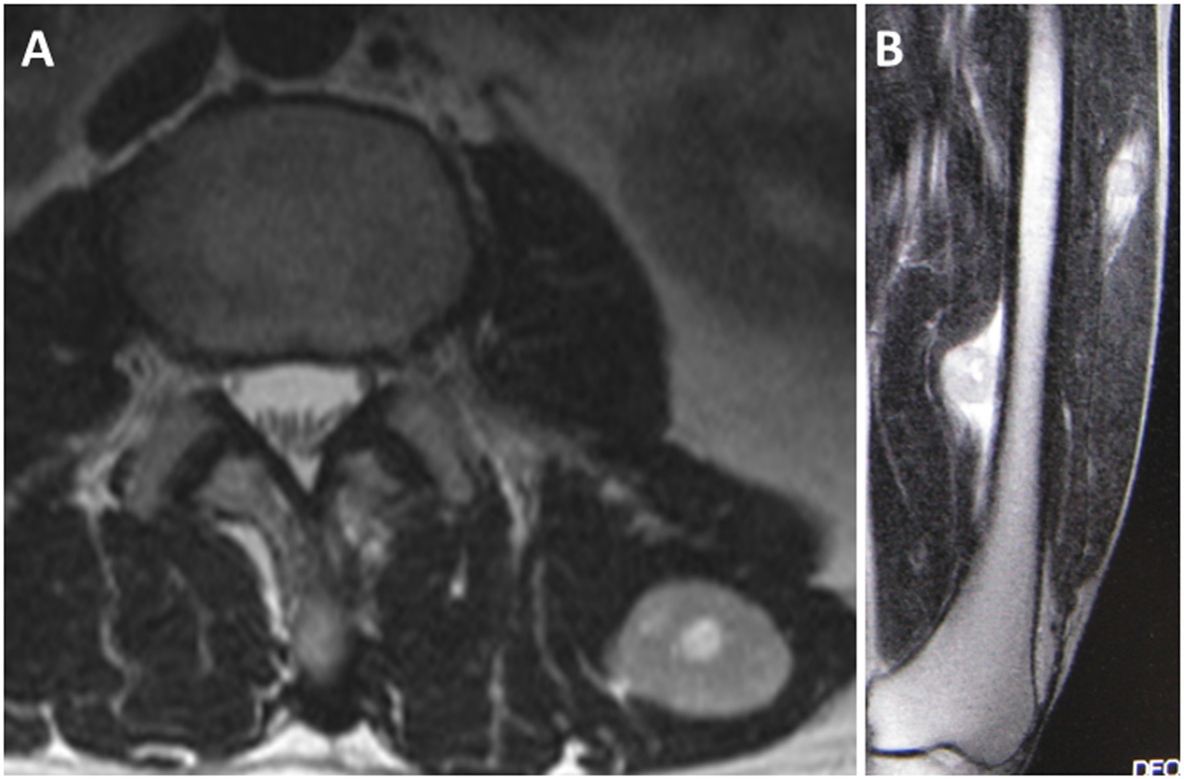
Magnetic resonance imaging findings of the paravertebral muscle and left thigh. Axial T2-weighted imaging reveals high-signal intensity masses in the paravertebral muscle (A) and the left quadriceps (B).
